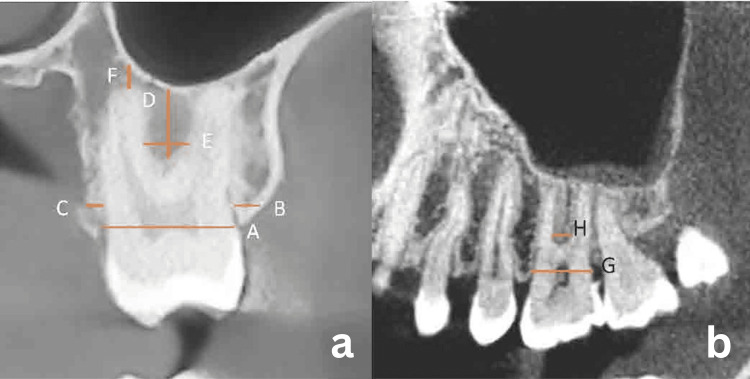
Maxillary molar measurement using CBCT scan a: coronal section. b: sagittal section. A: Buccolingual socket size. B: Width of the buccal bone. C: Width of the palatal bone. D: Distance from FMS to the furcation. E: Width of the interradicular bone 2 mm apical to the furcation. F: Distance from the root apex to FMS. G: Mesiodistal socket size. H: Width of the interradicular bone 2 mm apical to the furcation
